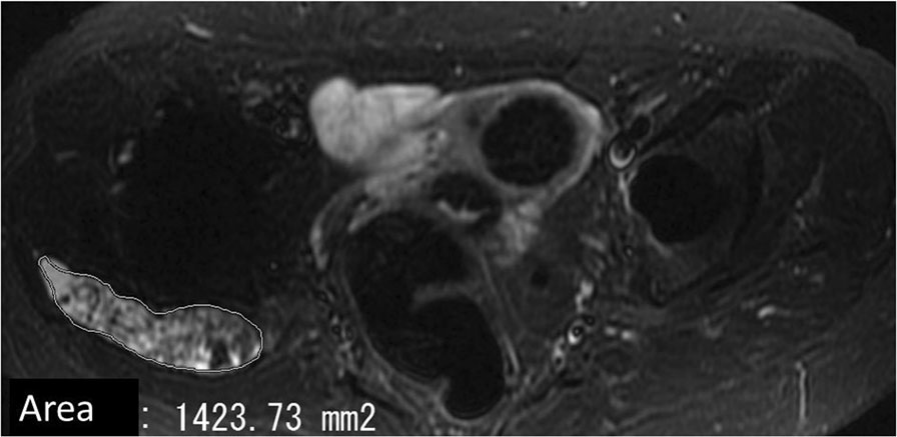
Measurement of pseudotumor size on a magnetic resonance image by manually outlining the greatest size of the mass using computer software
